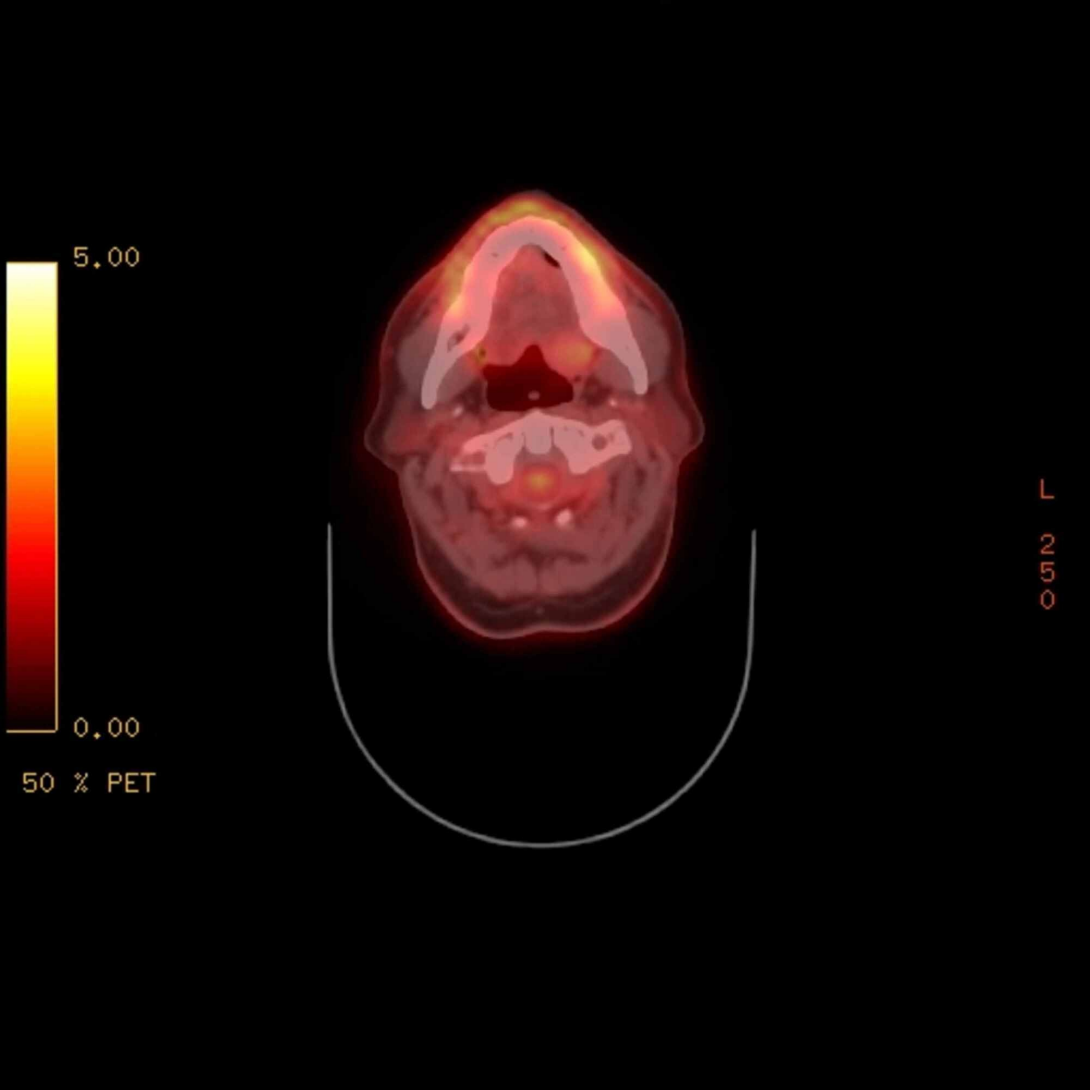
The left posterior tongue mass with increased uptake measuring about 1.5 cm x 0.6 cm (standardized uptake values (SUV) 2.6).
